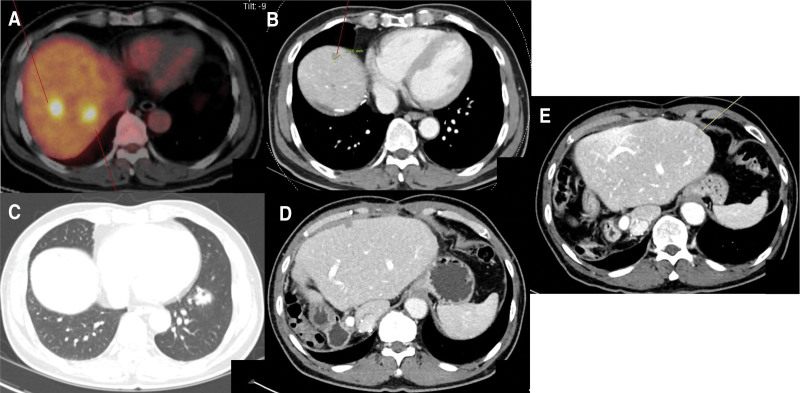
PET-CT or abdominal CT images. (A) Multiple metastases in the right lobe of liver in the PET-CT. (B) A metastatic nodule in the residual liver in the CT. (C) Metastatic nodules in both lower lobes in the CT. (D) A metastatic nodule in the residual liver (anterior subcapsular area) in the CT. (E) Hepatic metastasis in the left liver (subcapsular area) in the CT. CT = computed tomography, PET = positron emission tomography.
